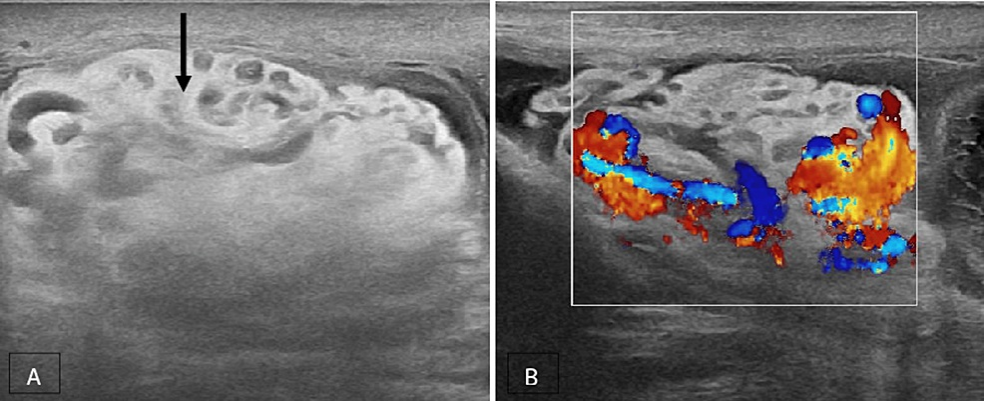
Funiculitis A, B Grey-scale and colour Doppler images of the right spermatic cord (solid black arrow) show enlarged, edematous, echogenic and heterogeneous spermatic cord with increased vascularity.
